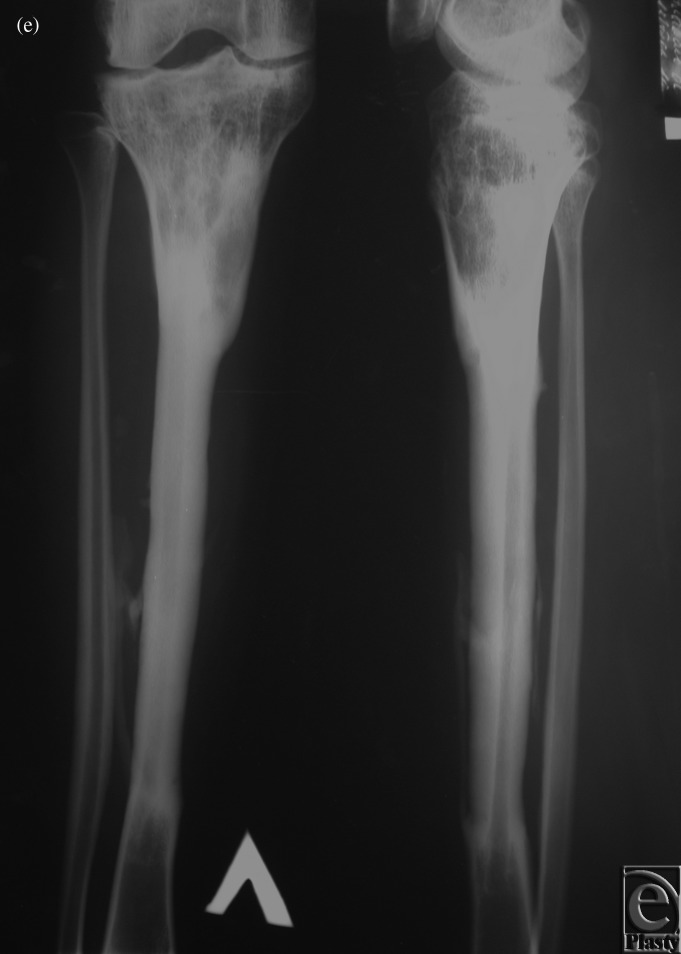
Case 3: Osteomyelitis of the lower leg. (*a*) Preoperative x-ray, (*b*) External fixation, (*c*) Fibula osteocutaneous flap, (*d*) The 2-year postoperative view, and (*e*) An x-ray 2 years after surgery.
